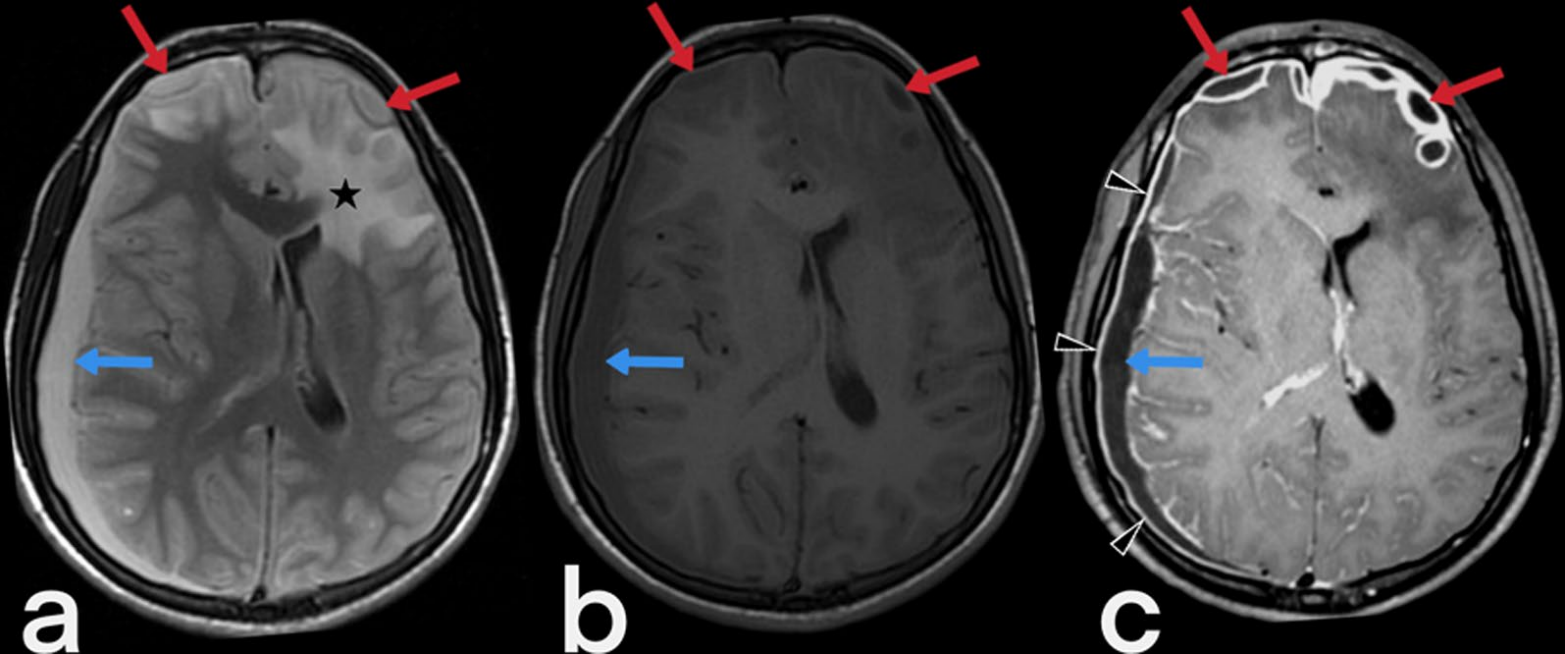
Axial FLAIR (a), pre-contrast (b) axial T1 weighted, and post-contrast (c) axial T1 weighted MR images of a 21-year-old male. Axial FLAIR image shows high signal intensity caused by edema and inflammation in the left frontal lobe (star). In both anterior frontal regions (red arrows) and right parietal area (blue arrows) are seen subdural abscesses. In addition, MR images demonstrate meningeal enhancement and thickening (arrowheads)
